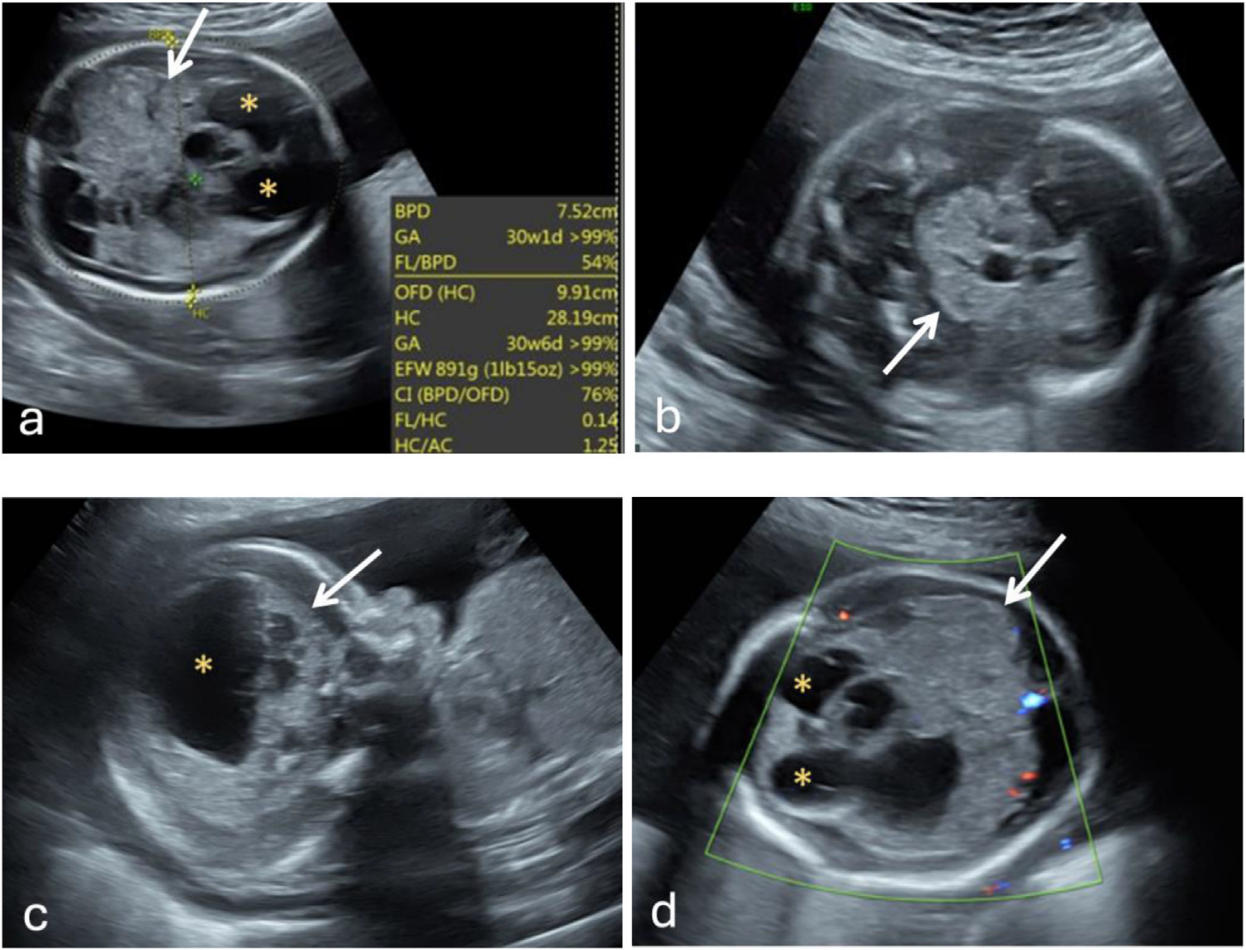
Detailed fetal anatomy ultrasound at 24 weeks and 0 days. Transverse (A, B, D) and sagittal (C) planes display architectural distortion within the cranial cavity. The supratentorial brain parenchyma has a markedly dysmorphic, heterogenous appearance (arrows) with dilated lateral ventricles (asterisks). Head circumference measurements (A) are consistent with a 30-week and 6 days gestation fetus, >99th percentile for gestational age. The relative lack of blood flow (D) in the region on 2D color flow mapping narrowed the differential to a complex evolving hemorrhage, ischemia, or mass.
